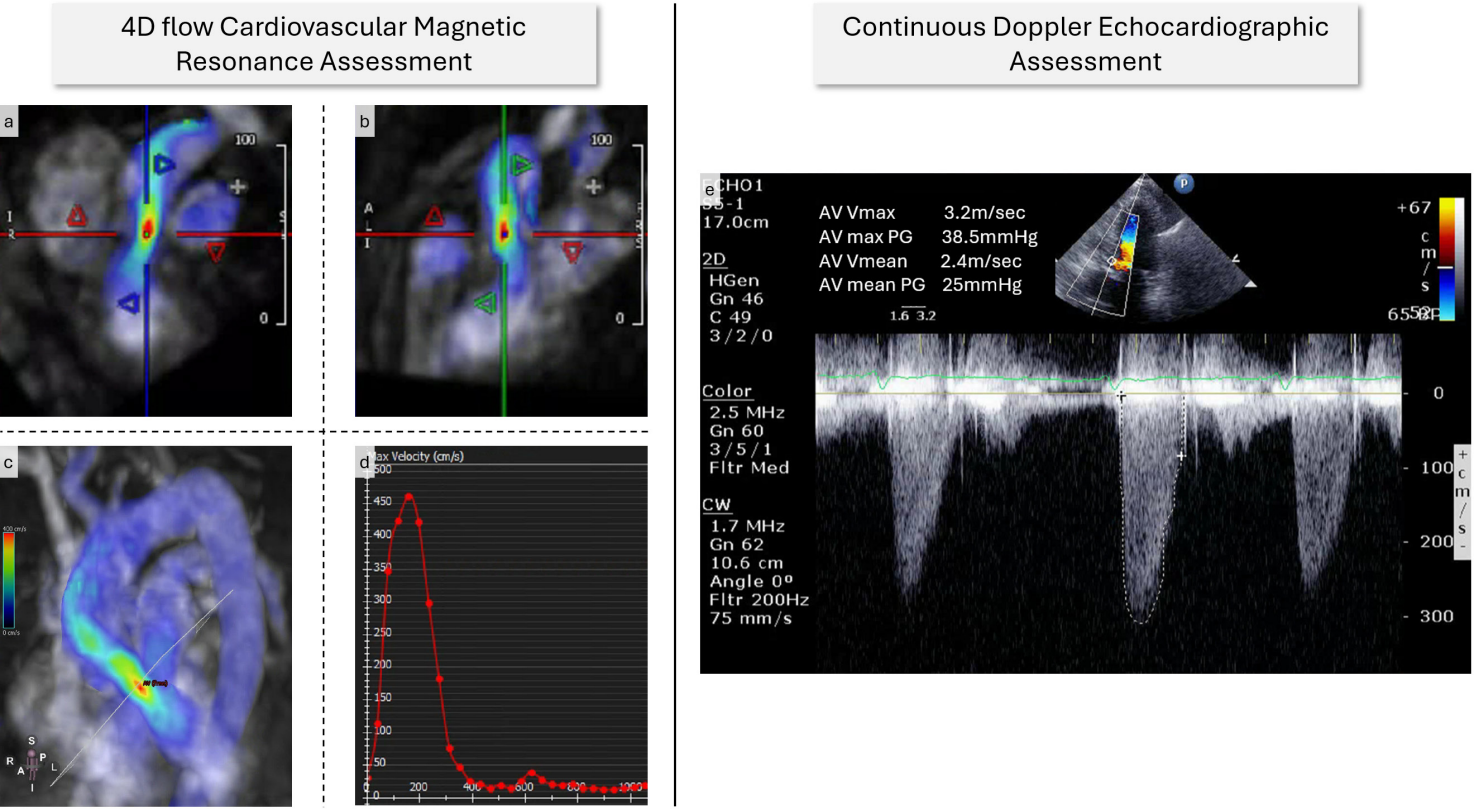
A case example from this study. (a–b) Demonstrates V_Peak_ assessment using 4D flow data superimposed on two orthogonal views of the aortic valve. (c) Three-dimensional visualisation of the plane where peak aortic valve velocity above 4 m/s can be identified. (d) 4D flow AV V_Peak_ was 4.7 m/s in this case. (e) CWD TTE assessment of the aortic valve stenosis with a V_Peak_ of 3.2 m/s. AV, aortic valve; CWD, continuous wave Doppler; TTE, transthoracic echocardiography; V_Peak_, peak aortic valve velocity.
